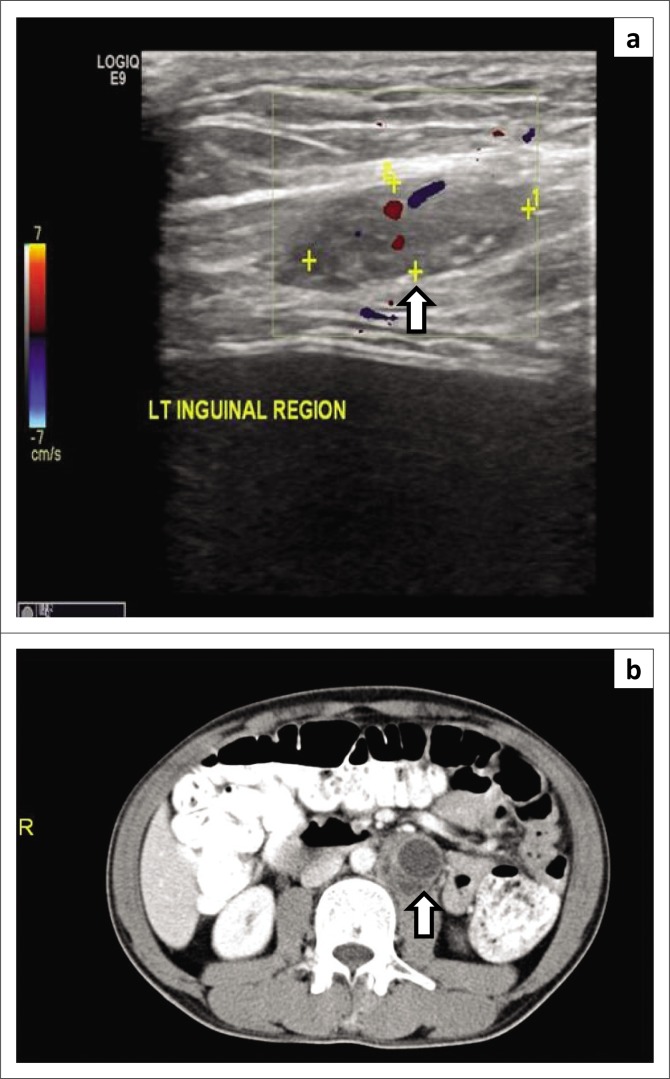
A 30-year-old male patient with a left-sided undescended testis: (a) Ultrasound imaging in the left inguinal location showed an atrophic and echogenic testis (bold white arrowhead). Postoperative findings were consistent with testicular non-seminomatous germ cell tumour and epididymis disjunction. (b) Contrast computed tomography for systemic work-up revealed necrotic heterogeneous lymph nodal metastasis (bold white arrow) in the left para-aortic location, which regressed after chemotherapy.
